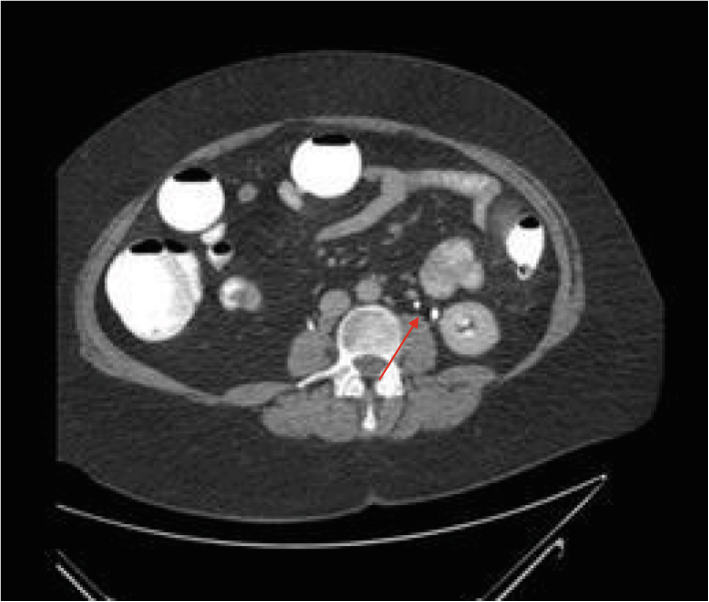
Preoperative axial view CT of the abdomen. Arrow indicates a possible left-sided double/bifid ureter in a duplex kidney around the L3 spinal level.
